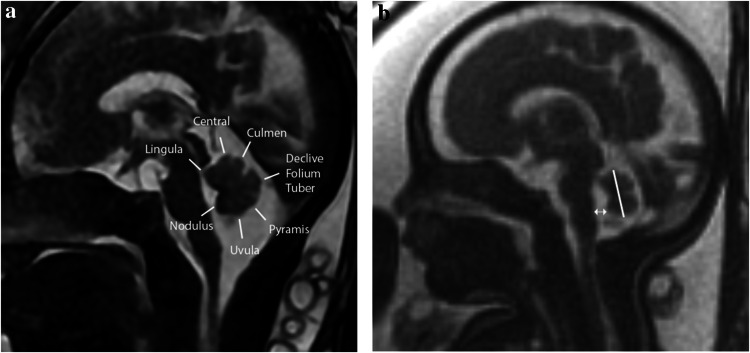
Vermian anatomy and isolated inferior vermian hypoplasia. a Fetal MRI performed on a 1.5-T scanner at 25 2/7 weeks delineates seven of nine cerebellar vermian lobules. b Fetal MRI midline sagittal T2 fast steady-state image performed at 31 1/7 weeks shows mild inferior vermian hypoplasia with the vermis craniocaudal dimension slightly smaller than expected for gestational age (*white line*). Inferior vermian distance is minimally widened, measuring 4 mm (*arrows*). Additionally, note the relatively equal amount of vermian tissue present above and below the fastigium-declive axis, whereas normally, the inferior vermis is slightly larger
